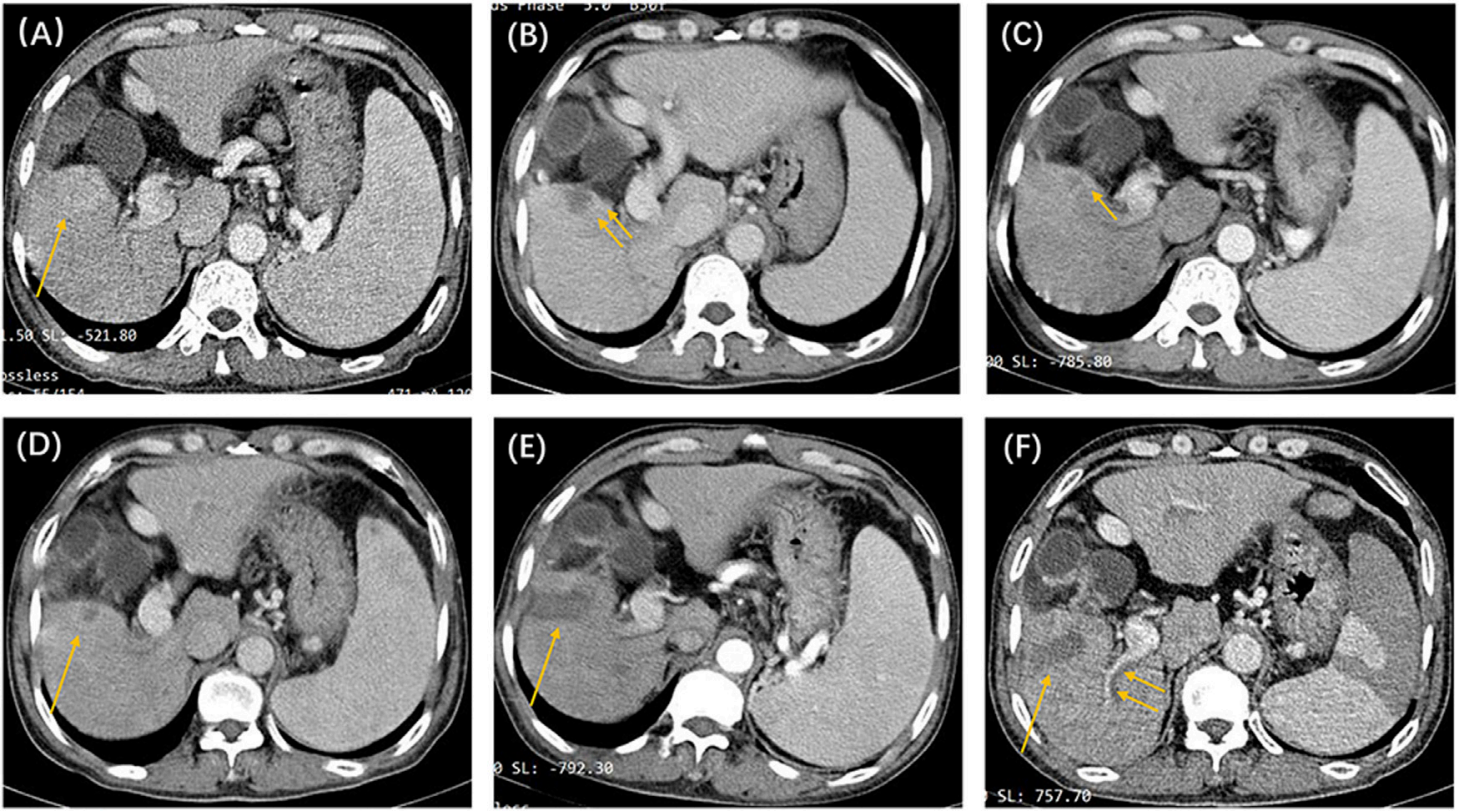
The patient was a 53-year-old man, with a clinical diagnosis of hepatocellular carcinoma, Barcelona Clinic Liver Cancer stage A, and pre-treatment hepatic function of the Child–Pugh grade (A). The above shows the contrast-enhanced CT images before and after locoregional interventional therapy. (A) Image taken before the initial drug-eluting beads–transcatheter arterial chemoembolization treatment, showing that the right lower liver lobe is full of blood-supplying, space-occupying lesions (single arrow). (B) Image taken 6 weeks after the initial TACE procedure. Suspicious nodular enhancement lesions could be seen around the target tumor (double arrows). The target tumor was assessed to be partial response based on modified response evaluation criteria in solid tumors (m-RECIST) criteria. (C) CT image 8 weeks after the second TACE procedure. Suspected annular enhancement was still seen at the margin (single arrow). The target tumor was assessed to be stable disease based on m-RECIST criteria. (D) Periodic image review 16 weeks after the second TACE procedure. Marginal enhancement and suspected recurrence (single arrow) could be seen. Tumor microwave ablation was performed under CT guidance. (E) Image taken 6 weeks after the ablation procedure. The target tumor was assessed to be complete response (single arrow) based on m-RECIST criteria. (F) Periodic image review 14 weeks after ablation. The filling defect seen in the right hepatic portal vein branch (double arrow) was considered to be a vascular invasion. According to the untreatable progression (UP) criteria, the patient was ultimately deemed to have UP.
